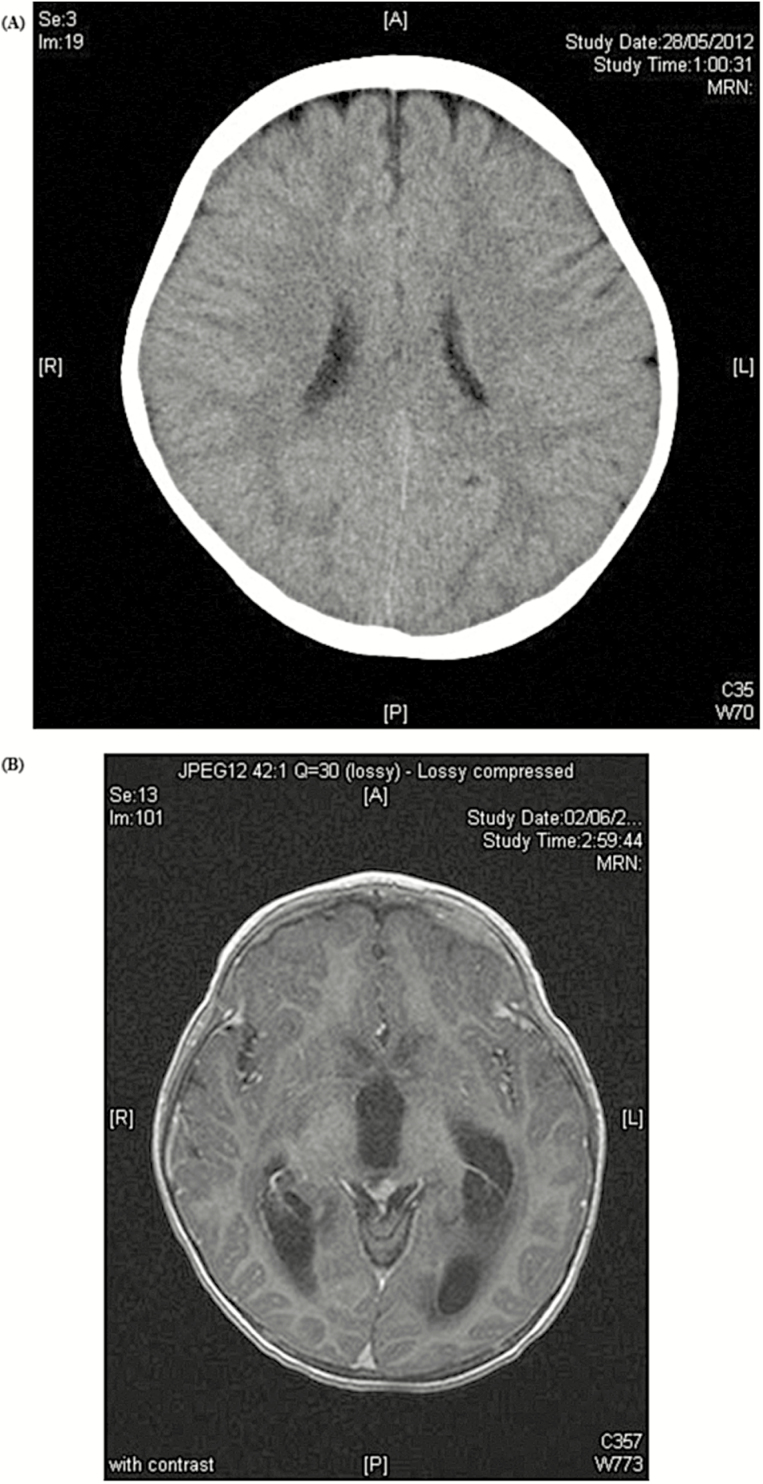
A*, Computed tomography of the brain performed on day 6 of illness showed no focal lesion and no displacement of midline structures, and the ventricles were not dilated. *B*, Magnetic resonance imaging of the brain on day 11 of illness showed moderate obstructive hydrocephalus with involvement of the lateral and third ventricles.
